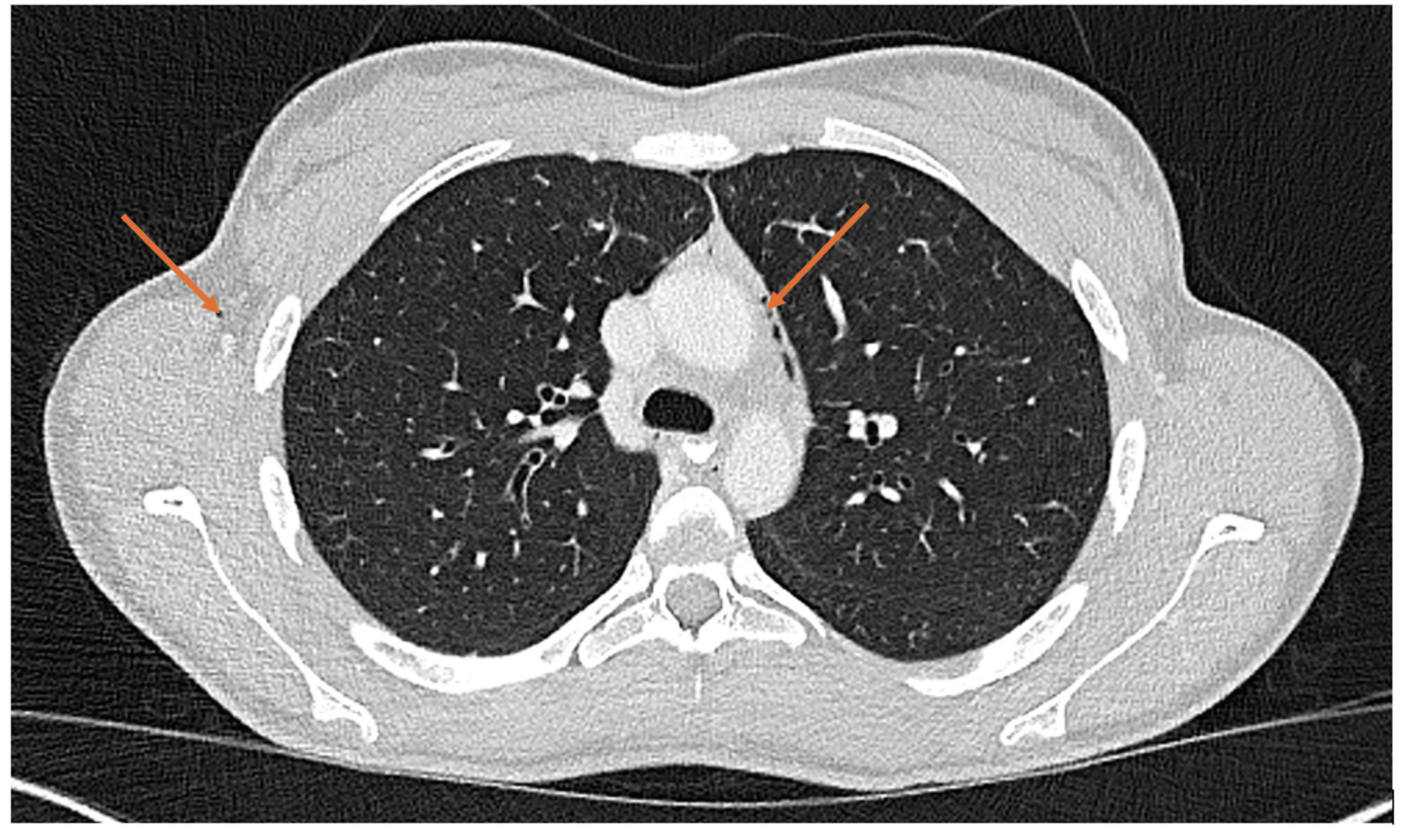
CT showing resolution of pneumorrhachis and pneumomediastinum CT: computed tomography
